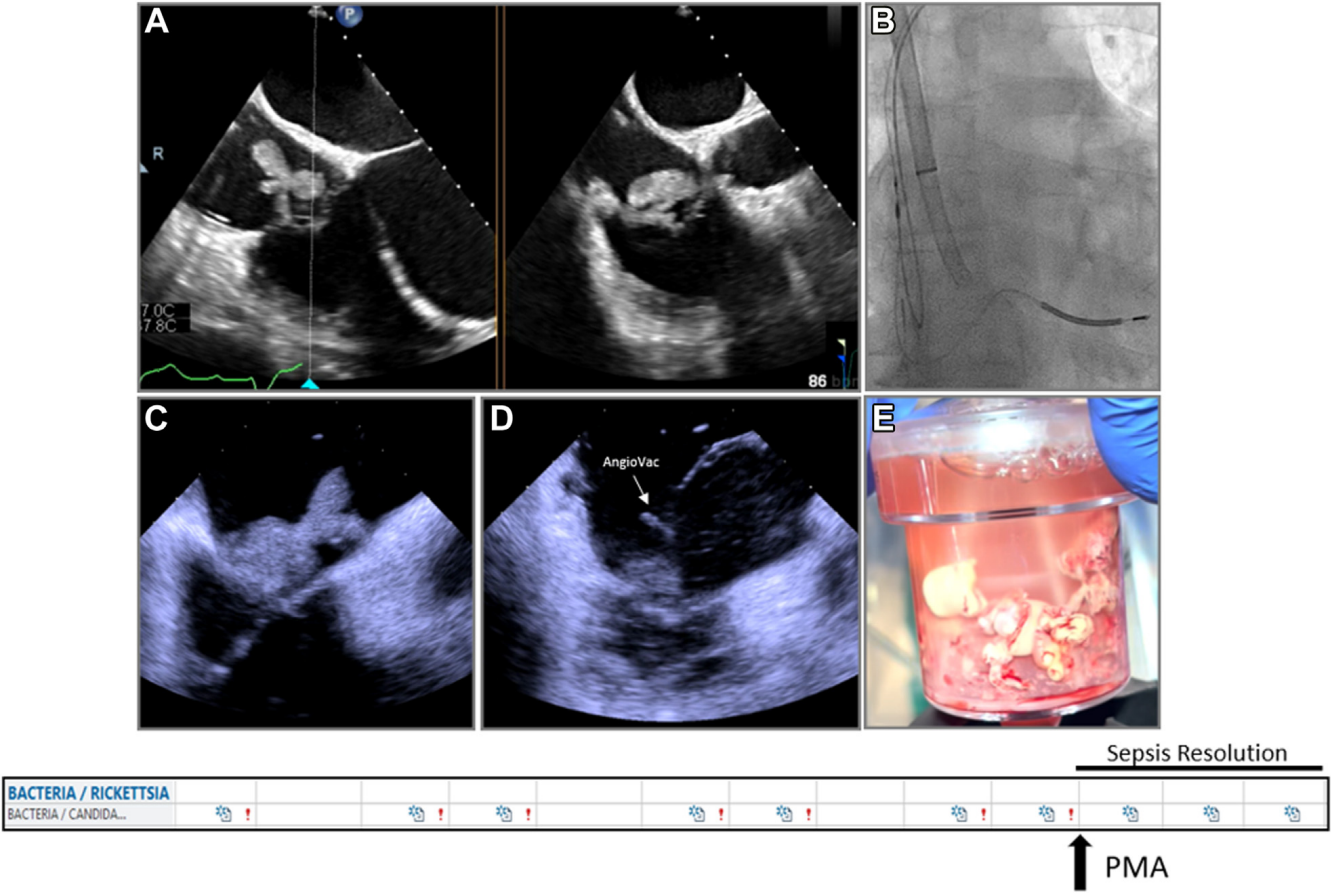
Percutaneous mechanical aspiration in persistent sepsis despite antimicrobials. Patient with large tricuspid valve vegetation (A) with persistent sepsis despite antimicrobials underwent large-bore aspiration using AngioVac under fluoroscopic (B) and intracardiac echocardiography (C,D) with effective debulking (E) and resolution of the sepsis.
